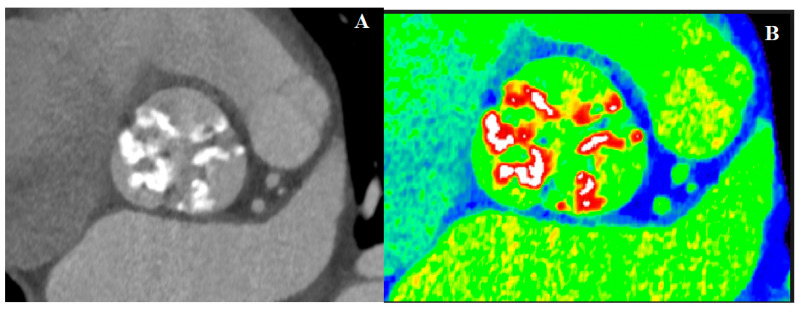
Measurement of aortic valve calcifications on representative images. (A) Low dose chest CT scan image focused on the aortic valve of an 86-year-old woman with low-flow low-gradient aortic stenosis and 9000 Agatston score. (B) In white color number in Hounsfield Units (HU) to quantify aortic valvular calcium on contrast-enhanced scans. Here, equal to 1000 HU. Courtesy of Marianna Adamo.
